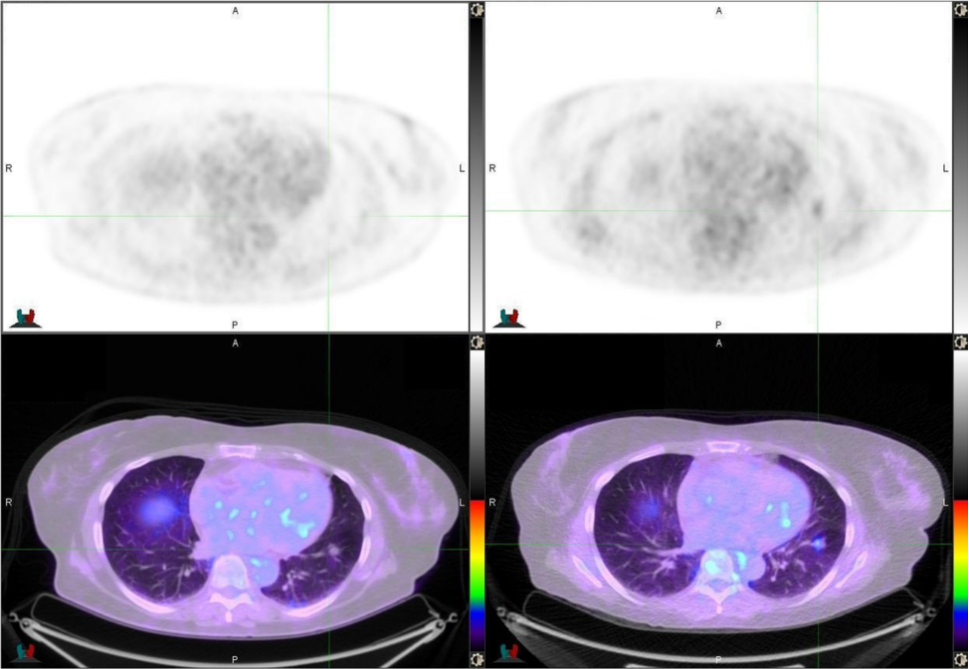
Lesion in the left lower lobe that was original classified on the WB-PET/CT (left column) as indeterminate and was subsequently changed to probably malignant on the 4D-PET/CT (right column). The SUVmax increased from 1.4 to 2.0. This lesion was subsequently confirmed as an adenocarcinoma.
